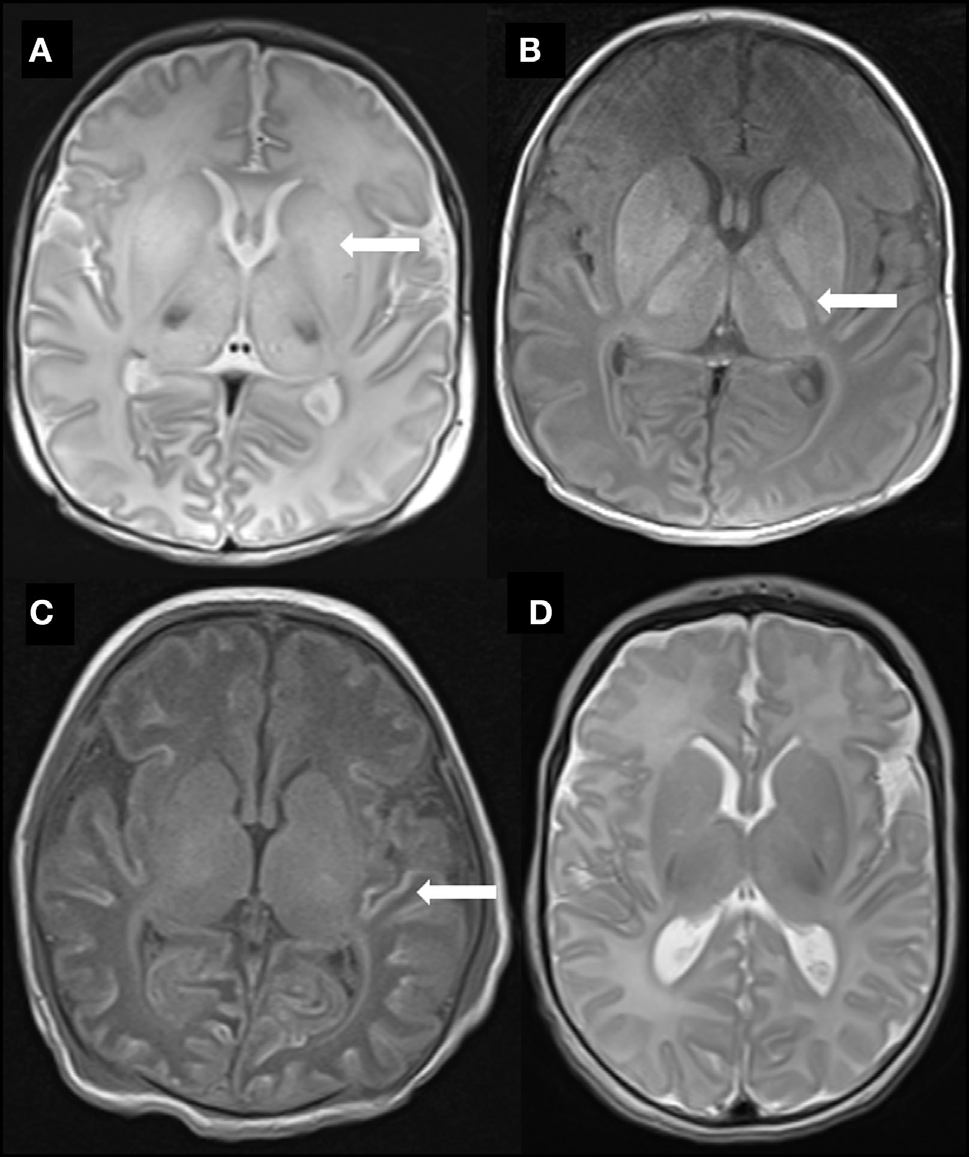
Representative MR images of study babies. (A) Diffuse high T2 signal throughout the basal ganglia (arrow) from baby 3. (B) Abnormal high T1 signal throughout the basal ganglia and thalami as well as complete loss of signal in the posterior limb of internal capsule (arrow) also from baby 3. (C) Widespread T1 high signal (arrow) in cortex (“cortical highlighting”) from baby 6. (D) Normal signal throughout the basal ganglia in T2-weighted image from baby 23.
